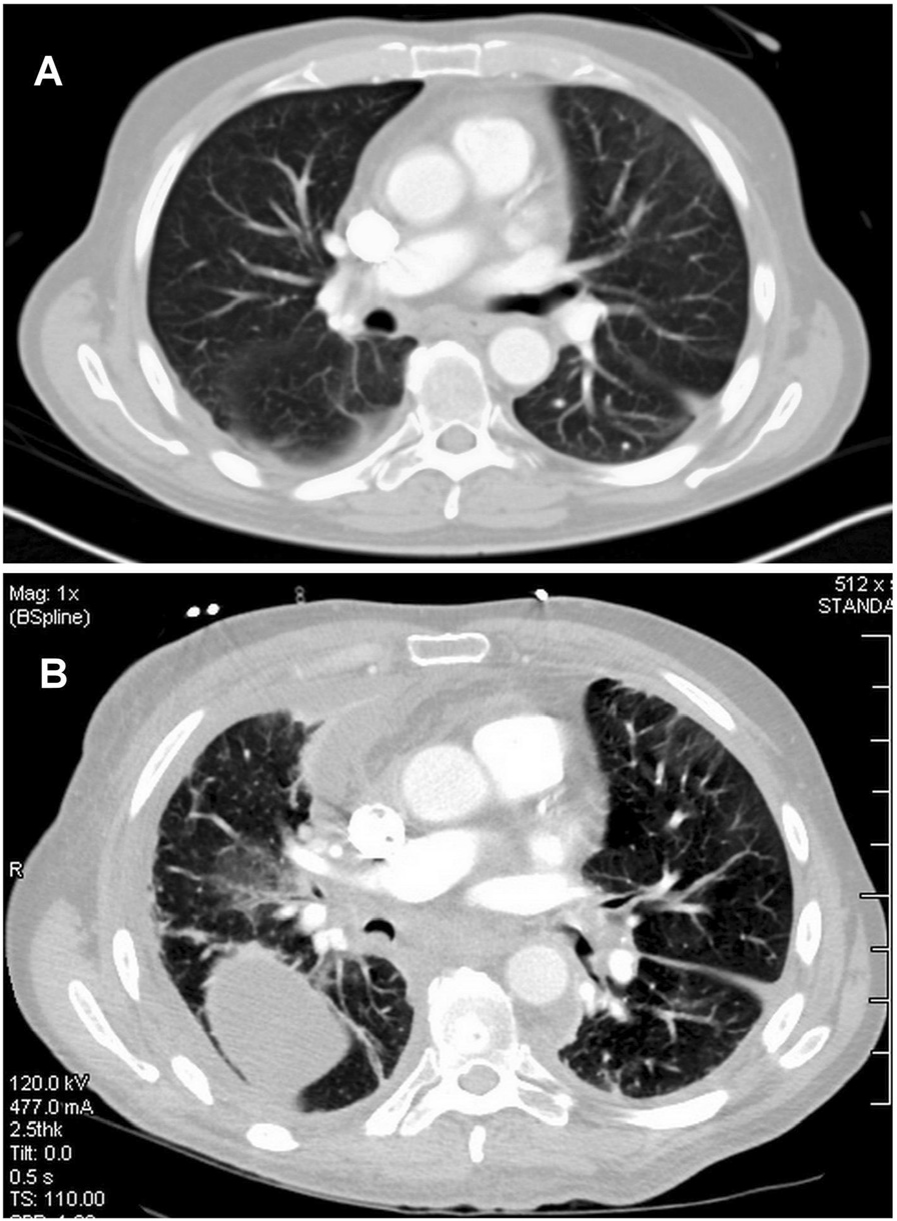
Computed tomography of the chest. Equivalent axial tomographic sections from November 2011 (panel A) and from December 2012 (panel B) are shown. Pericardial thickening measuring up to 6 mm is seen in the first tomogram. The interval development of circumferential pleural thickening and enlargement of the mediastinal shadow is evident in the second tomogram. The changes are more pronounced in the right pleural space, where there is a loculated effusion. Differences in the diameter of the large airways between the two tomograms may relate to differences in the respiratory cycle.
